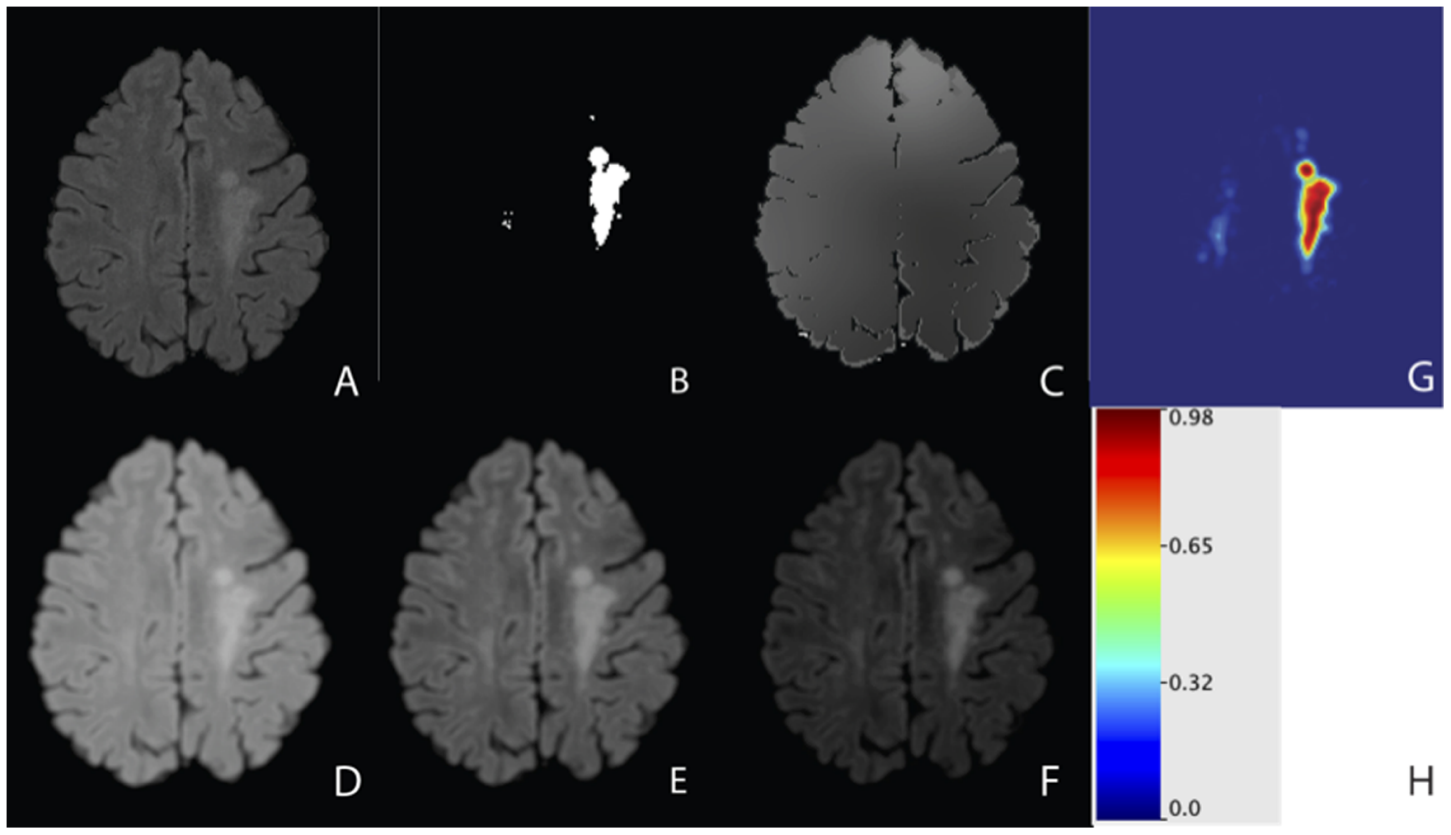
An axial slice from a single subject of the FLAIR volume with the normalization procedure, manual lesion segmentation, neighborhood functions of the FLAIR volume, and an example of a classification result. (A) FLAIR volume; (B) manual lesion segmentation; (C) FLAIR smoothed volume with a neighborhood n = 41; (D) FLAIR first local moment volume with neighborhood n = 5; (E) FLAIR second local moment volume with neighborhood n = 5; (F) FLAIR third local moment volume with neighborhood n = 5. The smoothed volumes act on large neighborhoods, while the local moment volumes act over smaller neighborhoods; (G) The probability map produced for the logistic regression classifier on the smoothed feature vector; (H) The scale of intensities in the probability map.
